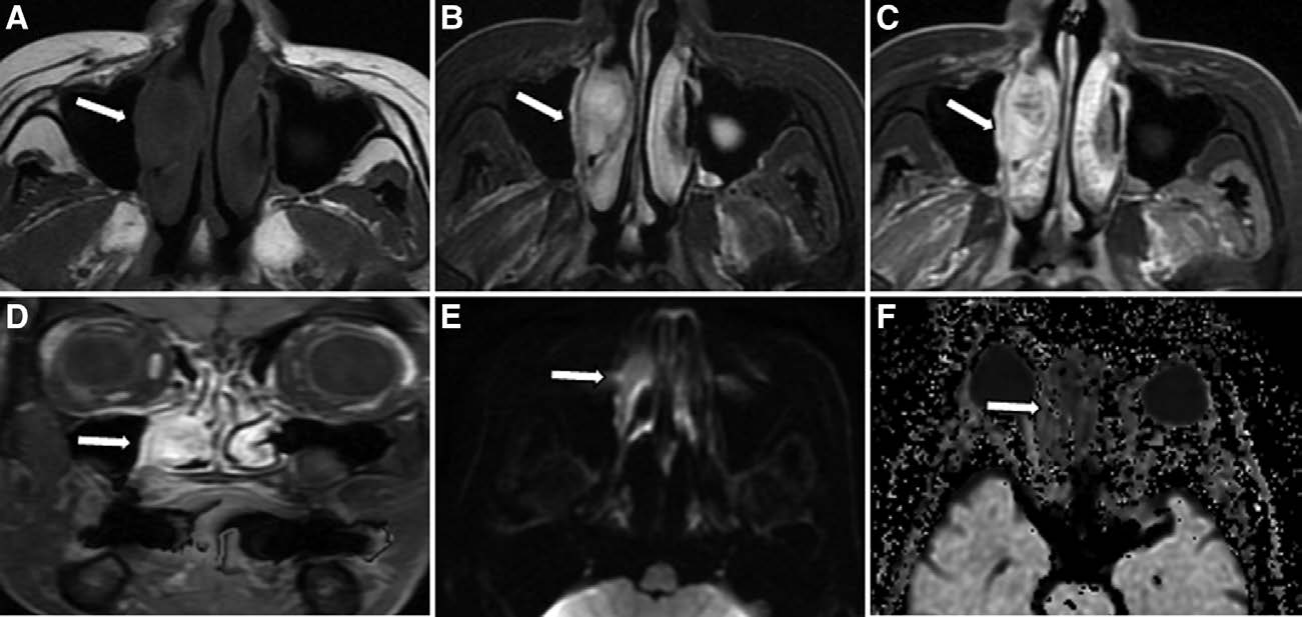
MRI showed that the tumor was located in the right nasal cavity. (A, arrow) T1WI sequence showed that the lesion was hypo-to-isosignal, about 2.7 cm × 1.6 cm in size. (B, arrow) T2WI sequence shows hypersignal. (C, axial, D, coronal, arrows) Contrast-enhanced T1WI showed obvious enhancement of the lesion and dilatation of the surrounding bone. (E, arrow) DWI showed limited spread of the lesion, showing high signal and (F, arrow) a low ADC. ADC = apparent diffusion coefficient, DWI = diffusion weighted imaging, MRI = magnetic resonance imaging, T1WI = T1-weighted imaging.
